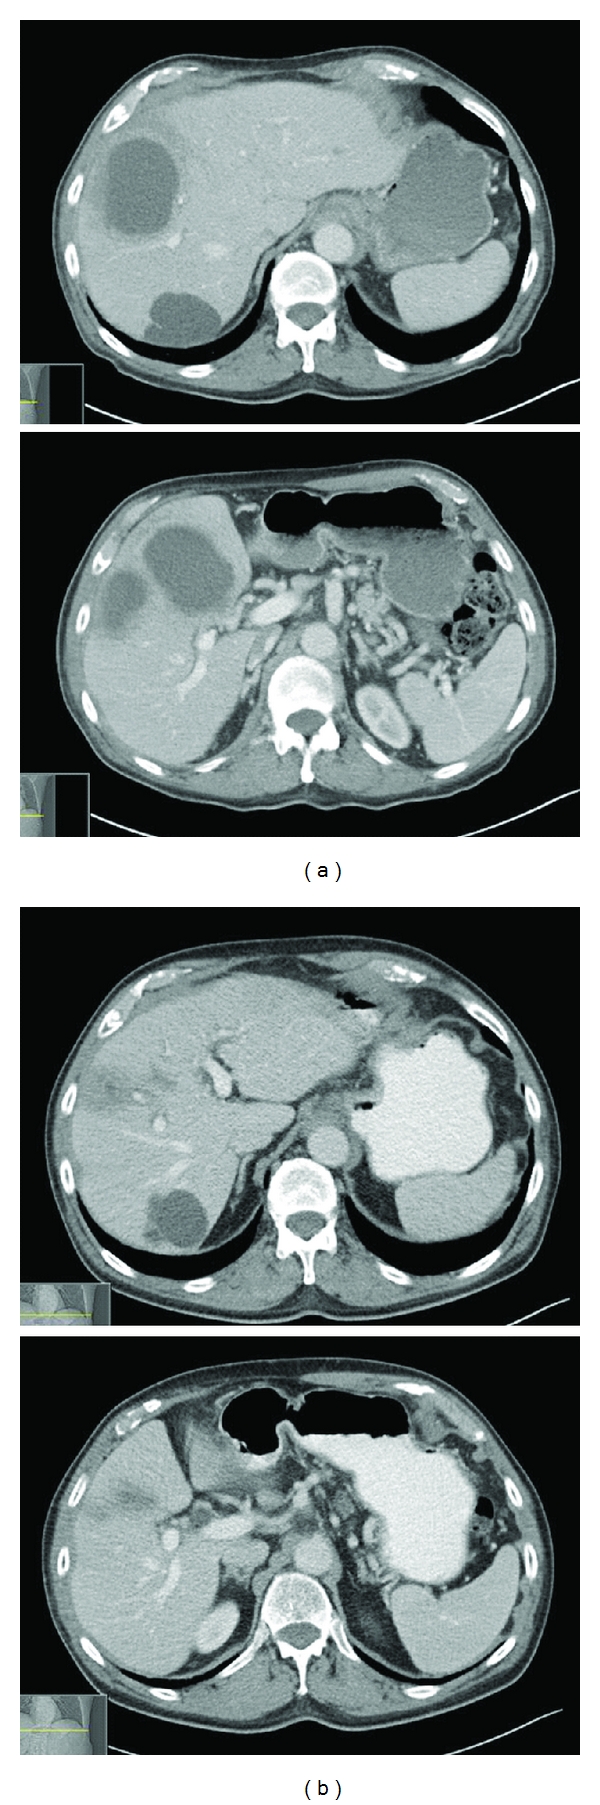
Abdominal CT images showing multiple liver abscesses before treatment (a) and resolution after treatment (b). Of note, patient has a benign liver cyst posteriorly that remained unchanged.
